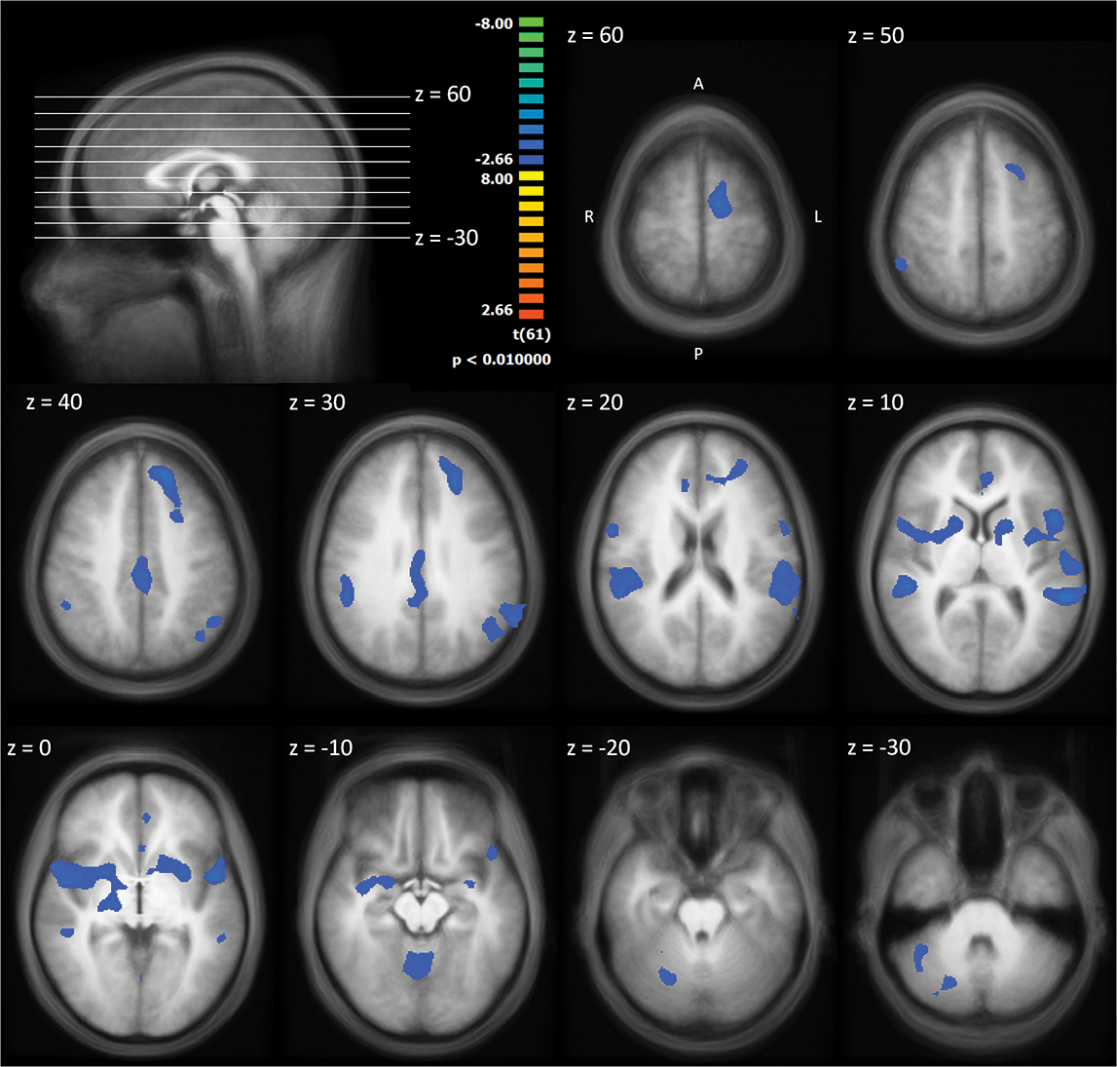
One sample t-test against zero for the slopes of the BOLD response (pain pictures > neutral pictures) across the whole sample. The statistical maps are shown superimposed on the averaged T1-weighted dataset of all subjects. Blue/green colours signify negative slopes of the BOLD response significantly different from zero, i.e. neural habituation.
